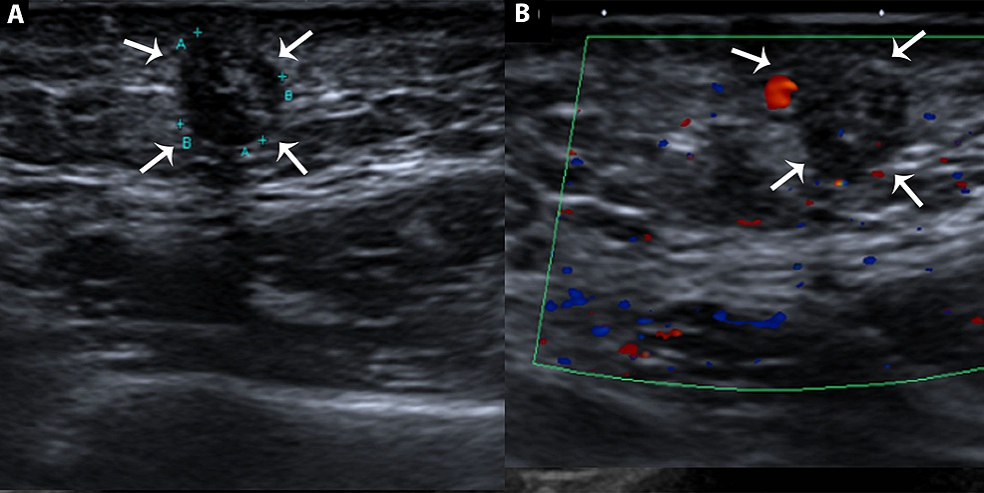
Ultrasound of cheek (A) Greyscale ultrasound of cheek showing an oval-shaped, well-defined isoechoic lesion, having hypoechoic margins (peripheral halo) and internal calcifications mainly in the peripheral regions (white arrows). It is associated with focal thinning of subcutaneous fat plains. (B) Doppler ultrasound of the cheek showing the absence of vascularity in the lesion (white arrows), consistent with the benign nature of the swelling. (A+ to A+ dimension of the lesion= 3 cm and B+ to B+ dimension of the lesion measures 2 cm).
